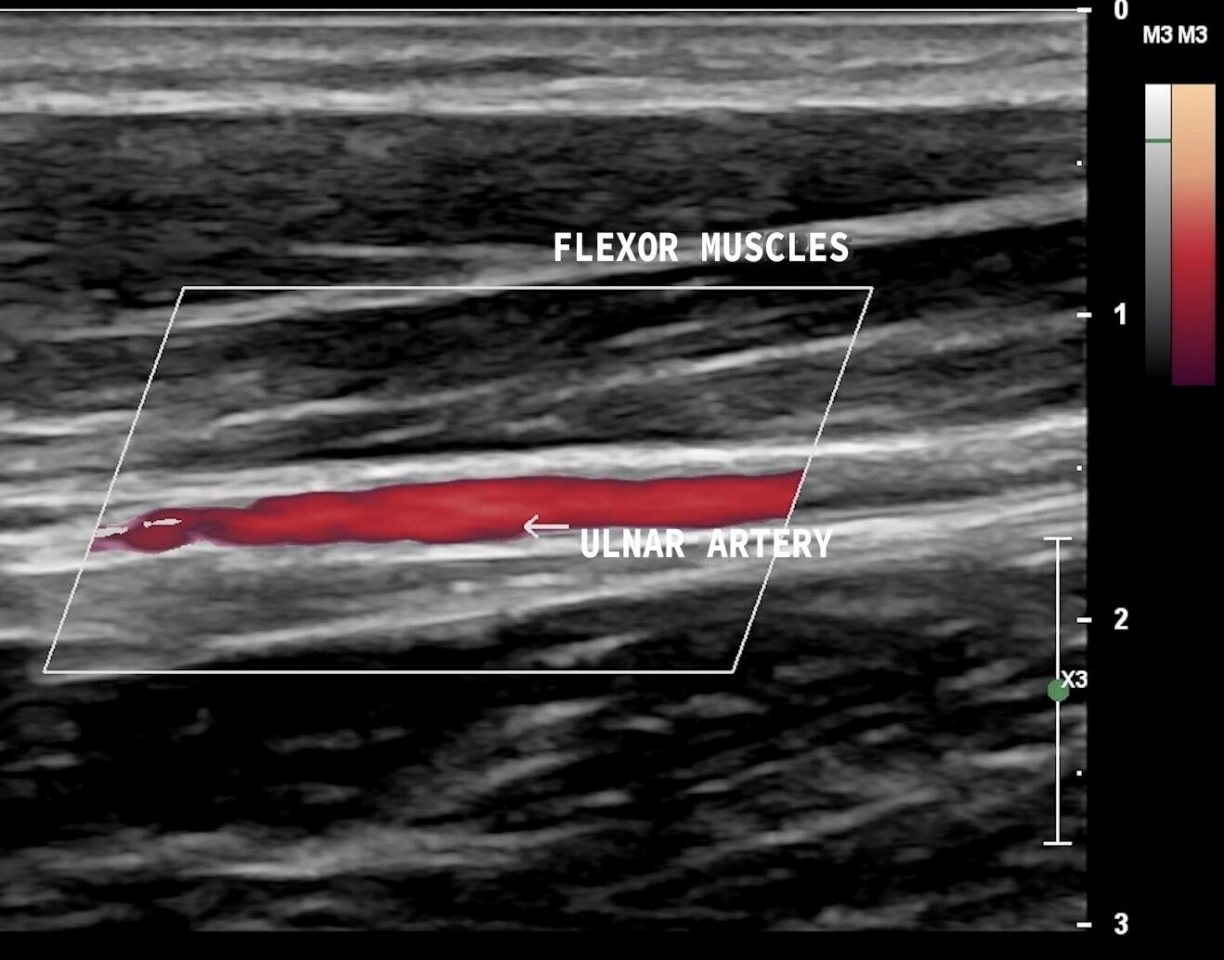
Vascular ultrasound, longitudinal view, with the ulnar artery following its normal path, coursing below the flexor muscles of the forearm.
